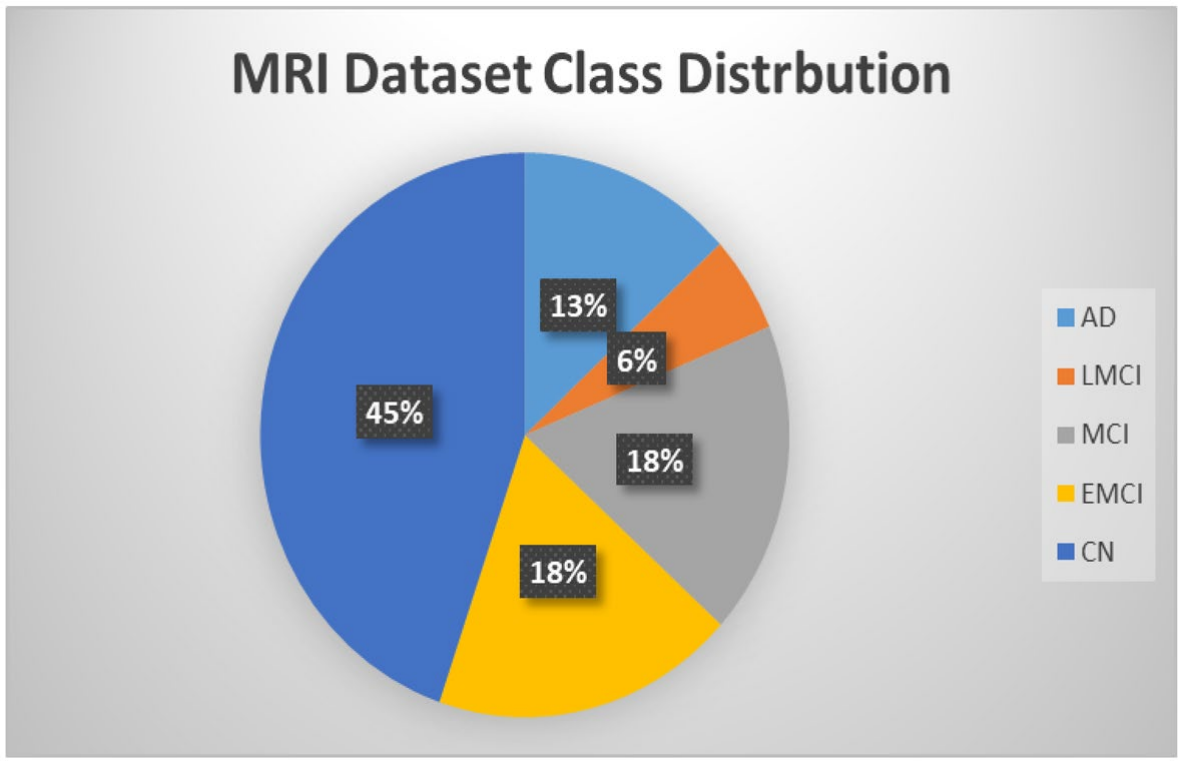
Class distribution of the MRI dataset.
